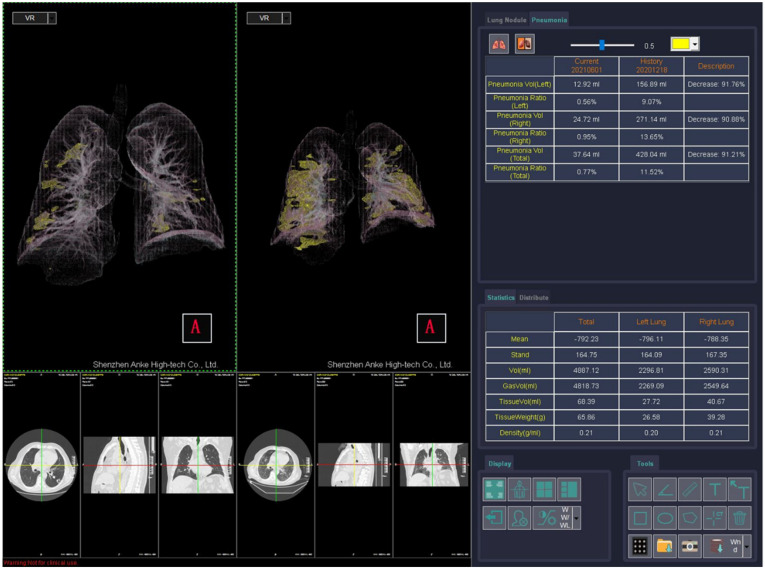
Automatic Segmentation of Thoracic Disease by COVID-19 using the pneumonia tool of ANKE ASG-340 CT workstation.
